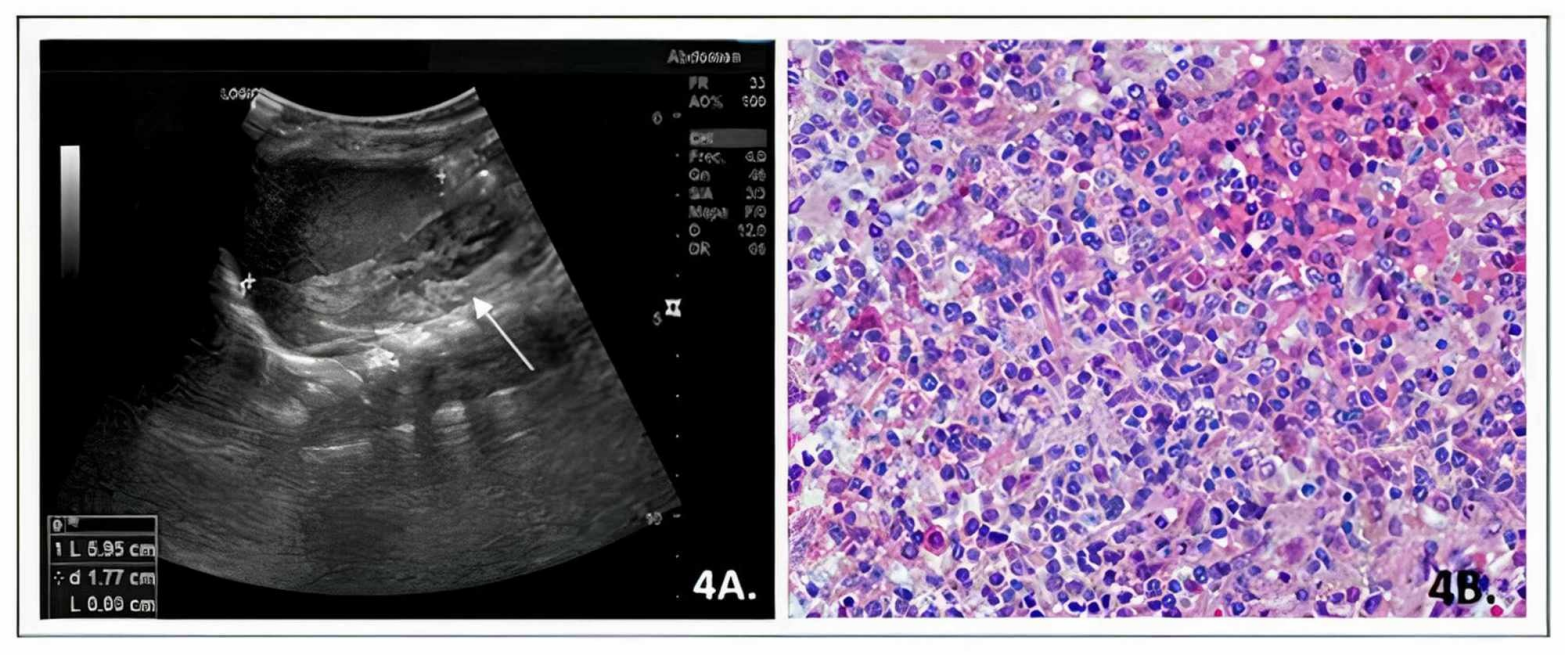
A. Total abdominal ultrasound with a slight parenchymal liver disease without hepatomegaly or splenomegaly; B. Bone marrow biopsy with infiltration by Langerhans cell histiocytosis
